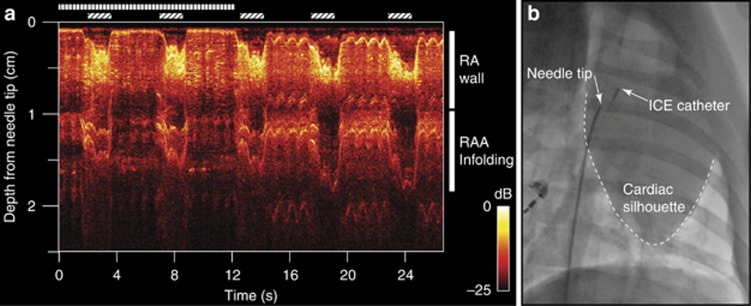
All-optical ultrasound imaging from the right atrium (RA) with depth scans shown longitudinally in time for M-mode imaging (a). The needle was pointed anteriorly and was initially held against the RA wall (vertical bars). Slight retraction was performed (>12 s), and pulsations of the RA wall became apparent (0.1–0.3 cm in depth from the needle tip). As mechanical ventilation was performed (diagonal bars), the resulting cardiac shifts produced changes in the apparent thickness of the RA wall. Right atrial appendage infolding and motion was visible beyond the RA wall. Conventional X-ray fluoroscopy imaging was performed concurrently (b).
